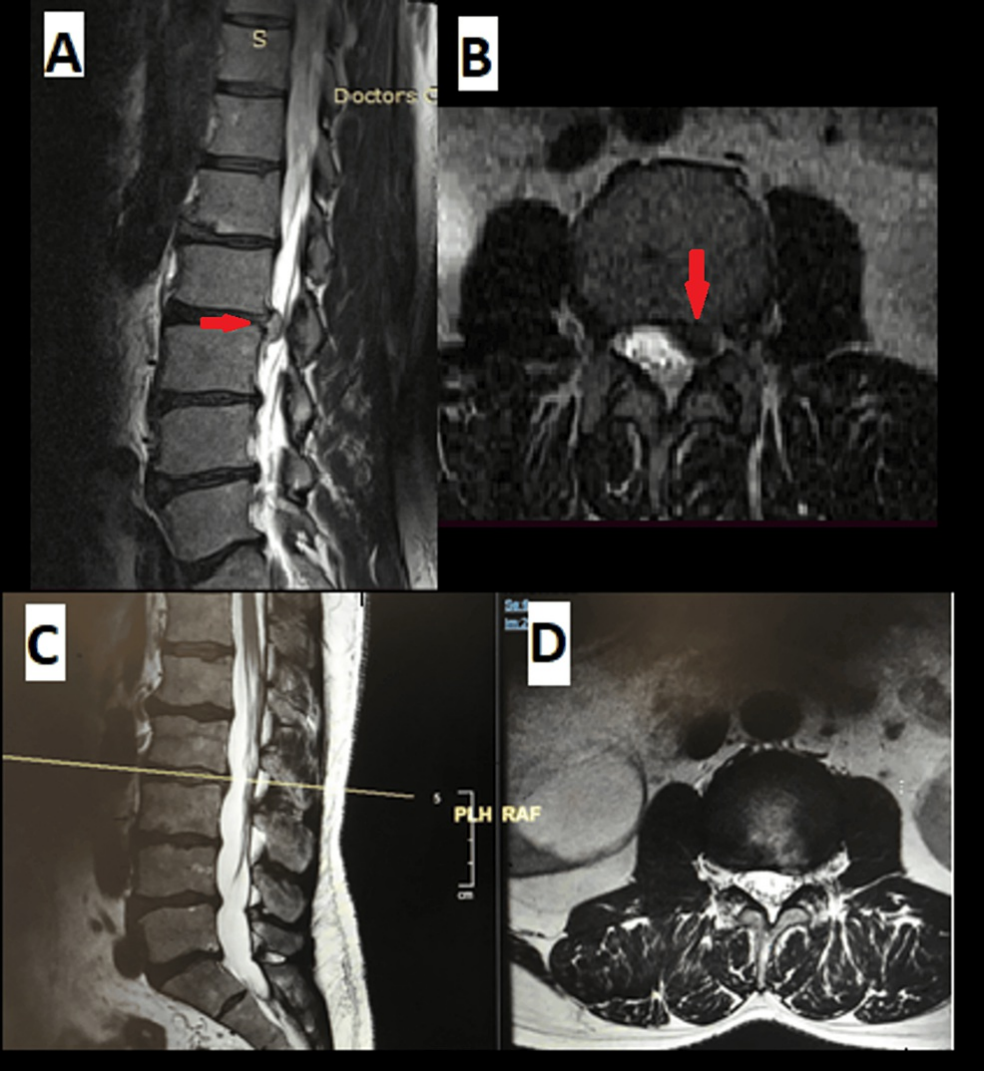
(A) Sagittal and (B) axial MRI of a patient two months post transforaminal endoscopic discectomy for L2-L3 left disc herniation, showing postoperative discal pseudocyst (red arrows). (C, D) Sagittal and axial MRI of the same patient treated conservatively successfully with complete resolution of the symptoms and disappearance of the cyst after three months.
